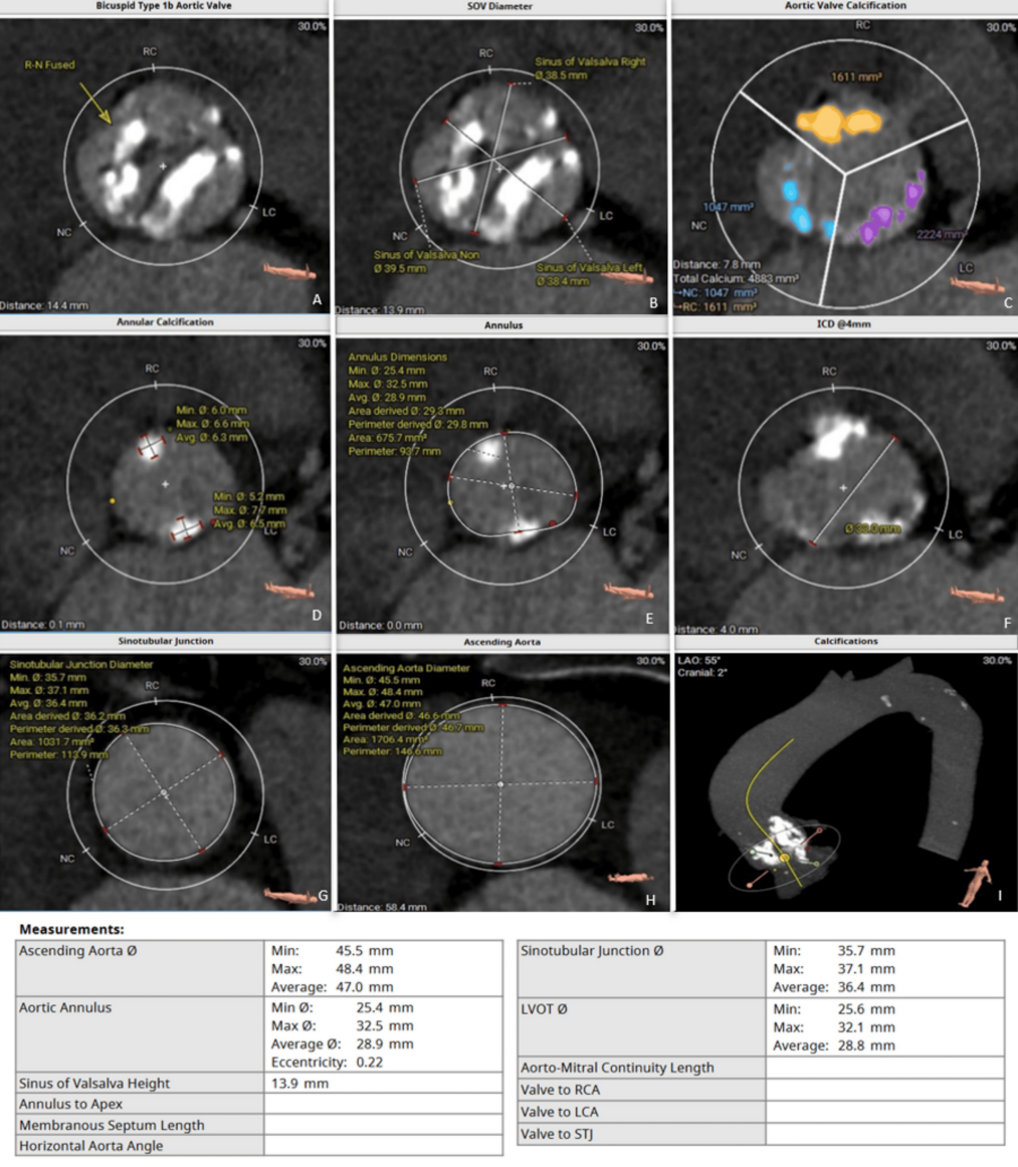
(A-L) Computed tomography angiography for the transcatheter aortic valve replacement protocol The findings reveal a bicuspid aortic valve type 1b, with severe calcification on the leaflets. These calcifications extend from the right coronary cusp and the left coronary cusp to the left ventricular outflow tract, significantly affecting the valvular anatomy. Additionally, the dimensions of the left ventricular outflow tract are comparable to those of the aortic annulus. A mild dilation of the ascending aorta is also noted, presenting some calcified atherosclerotic plaques. The aortic arch is displaced to the left and also shows the presence of atherosclerotic plaques. Finally, the supra-aortic trunks present atherosclerotic plaques, but no evidence of significant stenosis is found in the evaluated areas.
